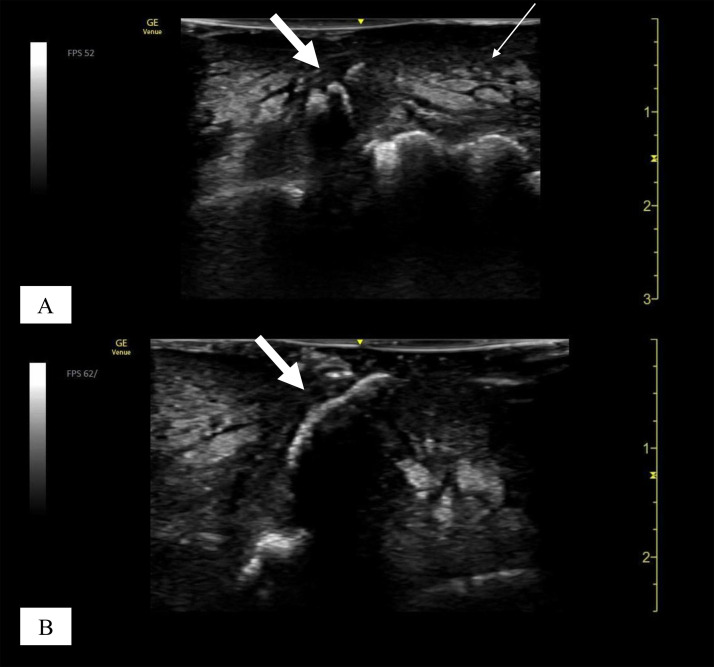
Ultrasound images of larvae. (A) Within the subcutaneous layer, hyperechoic structures with posterior acoustic shadowing were visualised, consistent with the presence of larvae (thick white arrow). Adjacent tissue exhibited a cobblestone appearance (thin white arrow). (B) A longitudinal view of a larva was visualised within the subcutaneous layer (thick white arrow).
